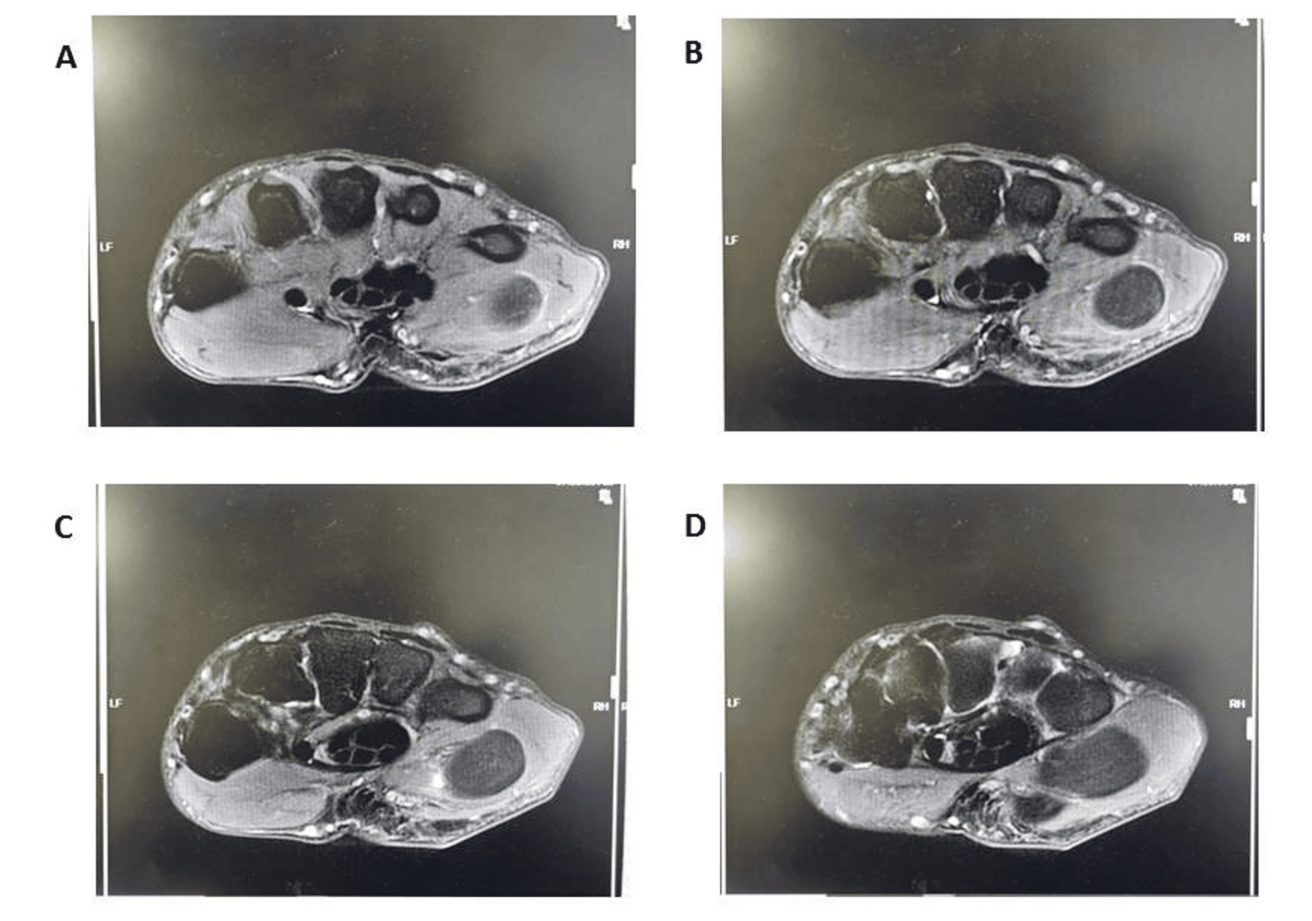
Sequential axial MRI images of the hypothenar region demonstrating a well-circumscribed, hyperintense soft tissue mass on T1-weighted sequences, consistent with lipoma
